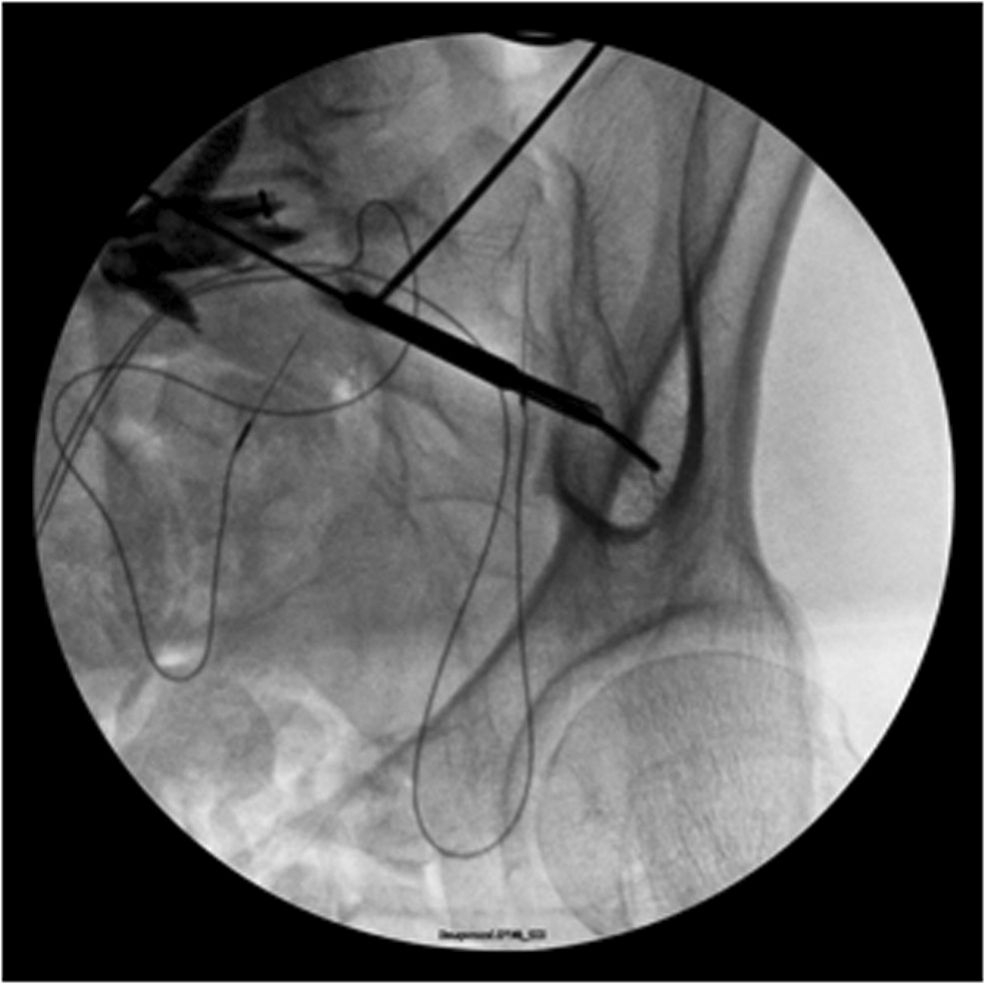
The radiographic teardrop that represents the radiographic confluence of the posterior superior iliac spine, the sciatic notch, and the anterior inferior iliac spine.
